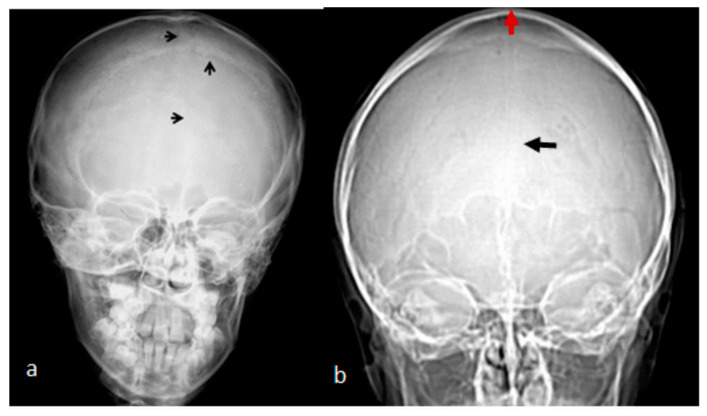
(a). AP skull radiograph of a 7-year-old boy with West syndrome showed facial asymmetry with early closure of the metopic suture (arrow head) similarly the coronal as well as the sagittal sutures showed post-closure sclerosis; (b) A 3D reformatted frontal cranial CT of a 35-year-old mother clearly showed the closure of the metopic (arrow) and the sagittal sutures causing a mid-sagittal bony bulge (red-arrow).
